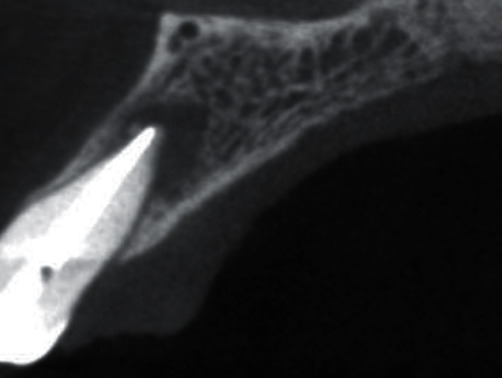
Postoperative CBCT showing healing of lesion on the labial aspect.
